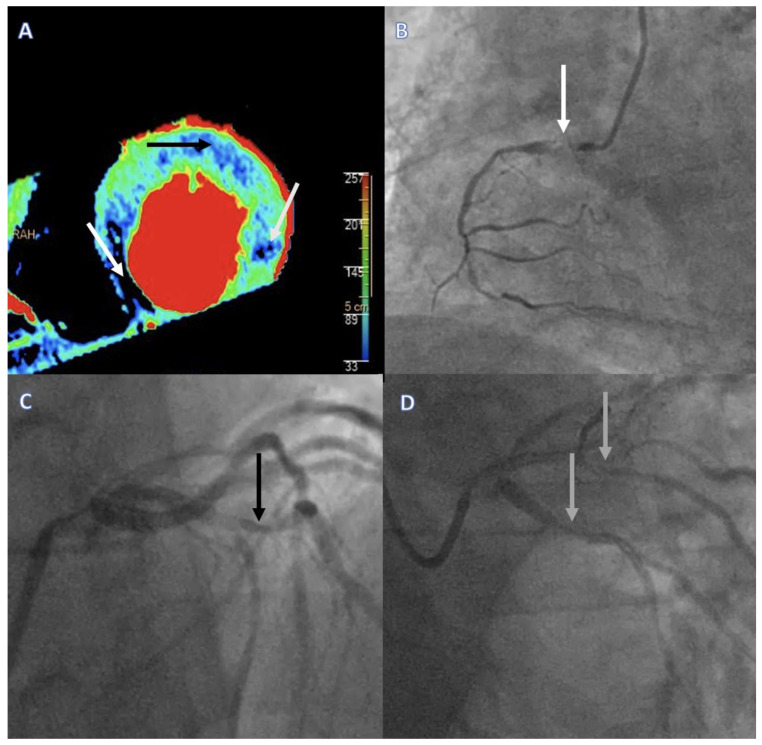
Regadenoson stress DPCT imaging results and corresponding invasive angiography and FFR findings in a multivessel CAD patient. (A) Regadenoson stress DPCT image depicting reduced myocardial blood flow (MBF < 101 mL/100 g/min) for all three main coronary territories. Necrosis was detected in the inferoseptal region (MBF 36 mL/100 g/min, white arrow), whereas ischemia was provoked in the anterior (MBF 69 mL/100 g/min, black arrow) and lateral (MBF 84 mL/100 g/min, gray arrow) wall of the LV. (B–D) Corresponding invasive coronary angiography images verifying a chronic total occlusion of the right coronary artery (white arrow), severe stenosis of the left anterior descending artery (FFR = 0.65) (black arrow), and severe stenosis of the circumflex (FFR = 0.75) and intermediary coronary arteries (gray arrows). DPCT = dynamic perfusion computed tomography, FFR = fractional flow reserve, MBF = myocardial blood flow, LV = left ventricle.
